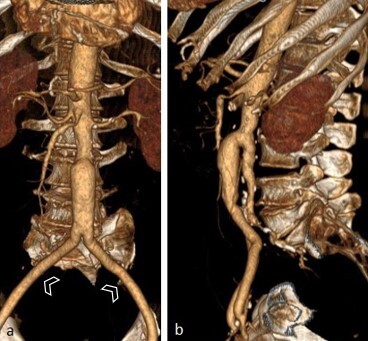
Serial volume-rendered CT images in a frontal view (a) and left lateral view (b), showing duplication of the inferior portion of the abdominal aorta, which bifurcates into the external iliac arteries (arrowheads) and the posterior portion, bifurcating into the internal iliac arteries (thrombosed in this study).
